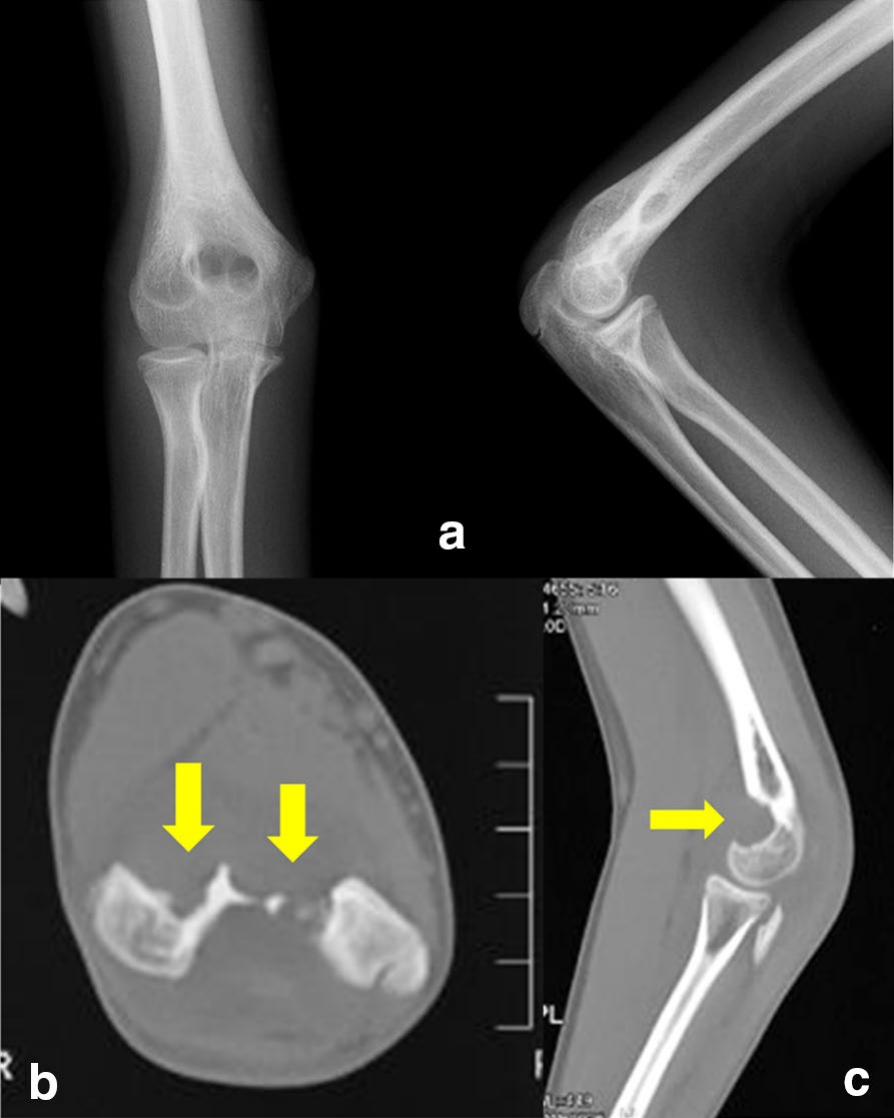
Plain radiography and computed tomography (CT) findings on admission. Plain radiography (a), axial CT (b) and sagittal CT (c) of the *right* elbow shows scalloping (*yellow arrow*) of the anterior portion of the distal humerus.
